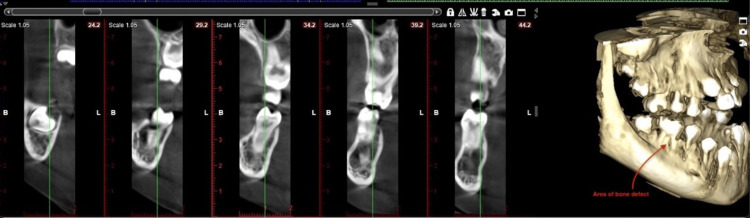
Cone-beam computed tomography image of grade II furcation involvement
